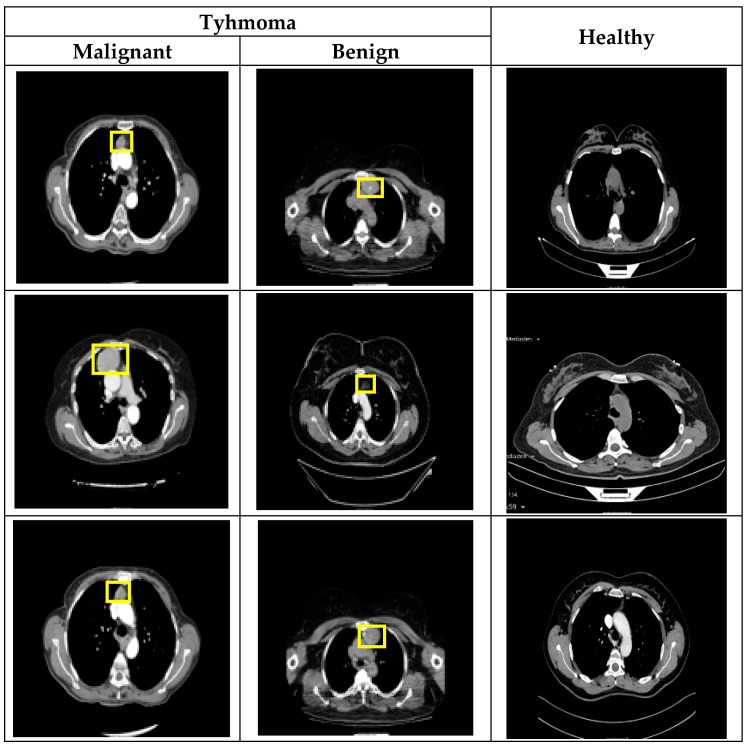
Example CT images from the dataset.
